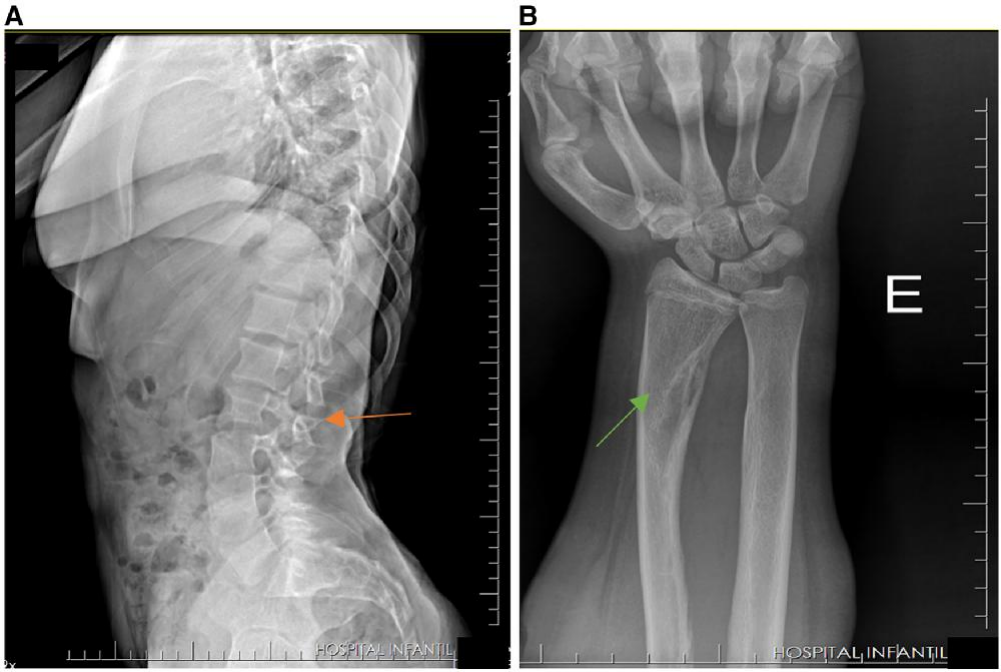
Radiographic images showing the patient aged 14 years and 10 months during use of burosumab. A, X-ray of the lumbar spine. Arrow indicates the first lumbar vertebra (L1), which is used as a reference point for assessing thoracolumbar dextroscoliosis; B, x-ray of the right wrist. Arrow indicates a cortical defect in the distal radius of the left wrist.
